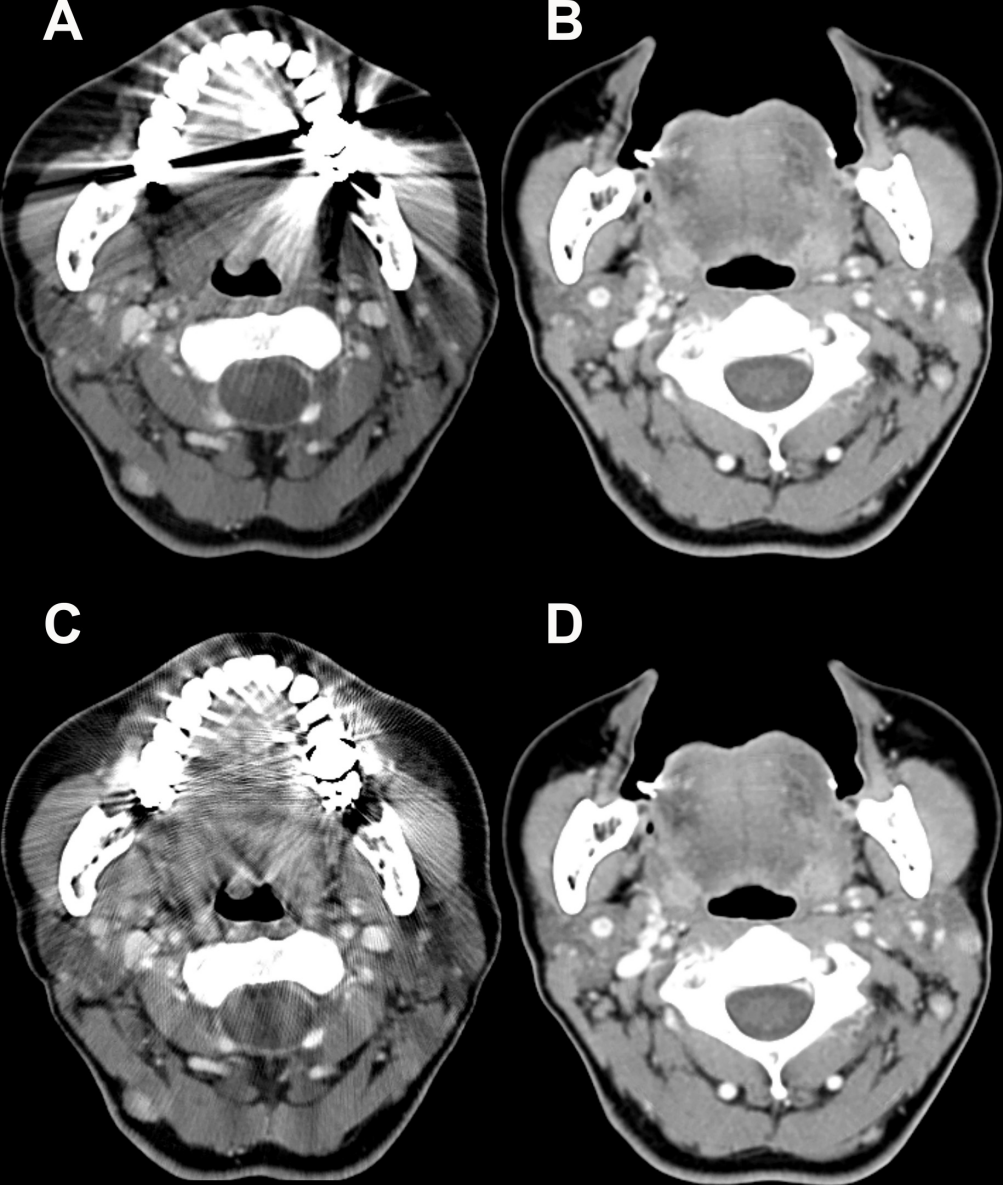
Post-contrast Computed Tomography (CT) images of a 56-year-old woman with a dental prosthesis. On the conventional CT image, the mobile tongue is barely seen due to the metallic artifact (A). However, the structure is clearly seen on the open mouth CT image (B), without overlapping dental prosthesis in the same imaging plane. (C) The additional application of O-MAR to conventional CT shows a substantial decrease in the extent of streak artifacts, but there are still remaining streaks. (D) On open mouth CT images with O-MAR processing, the added effect of O-MAR is not noticeable, since the streak artifact is already displaced from the open mouth maneuver. O-MAR = metal artifact reduction for orthopedic implants.
